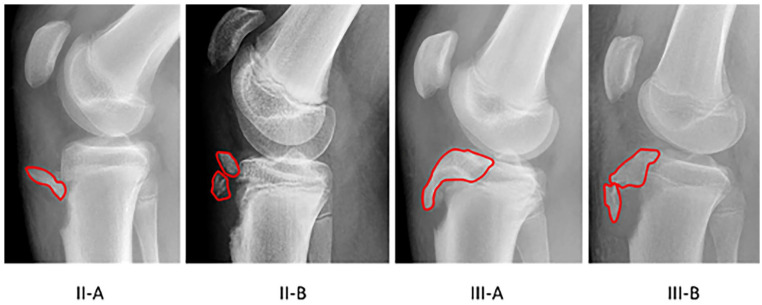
Representative preoperative lateral X-rays demonstrating the modified Ogden classification of tibial tubercle fractures. In a type I fracture (not depicted), the fracture line is through the secondary ossification center. In a type II fracture, the fracture occurs through the junction of the proximal tibia and tubercle ossification centers. Type II fractures can be either not comminuted (II-A) or comminuted (II-B). A type III fracture is a true Salter-Harris type III injury that is intra-articular. In subtype III-A, the tubercle and the anterior part of the proximal tibial epiphysis form a single unit. In subtype III-B, the fragment is comminuted, and the site of fragmentation is at the junction of the ossification centers of the proximal end of the tibia and the tubercle. A type IV fracture (not depicted) extends posteriorly through the entire proximal tibial physis, creating an avulsion of the entire epiphysis.^7,28^
